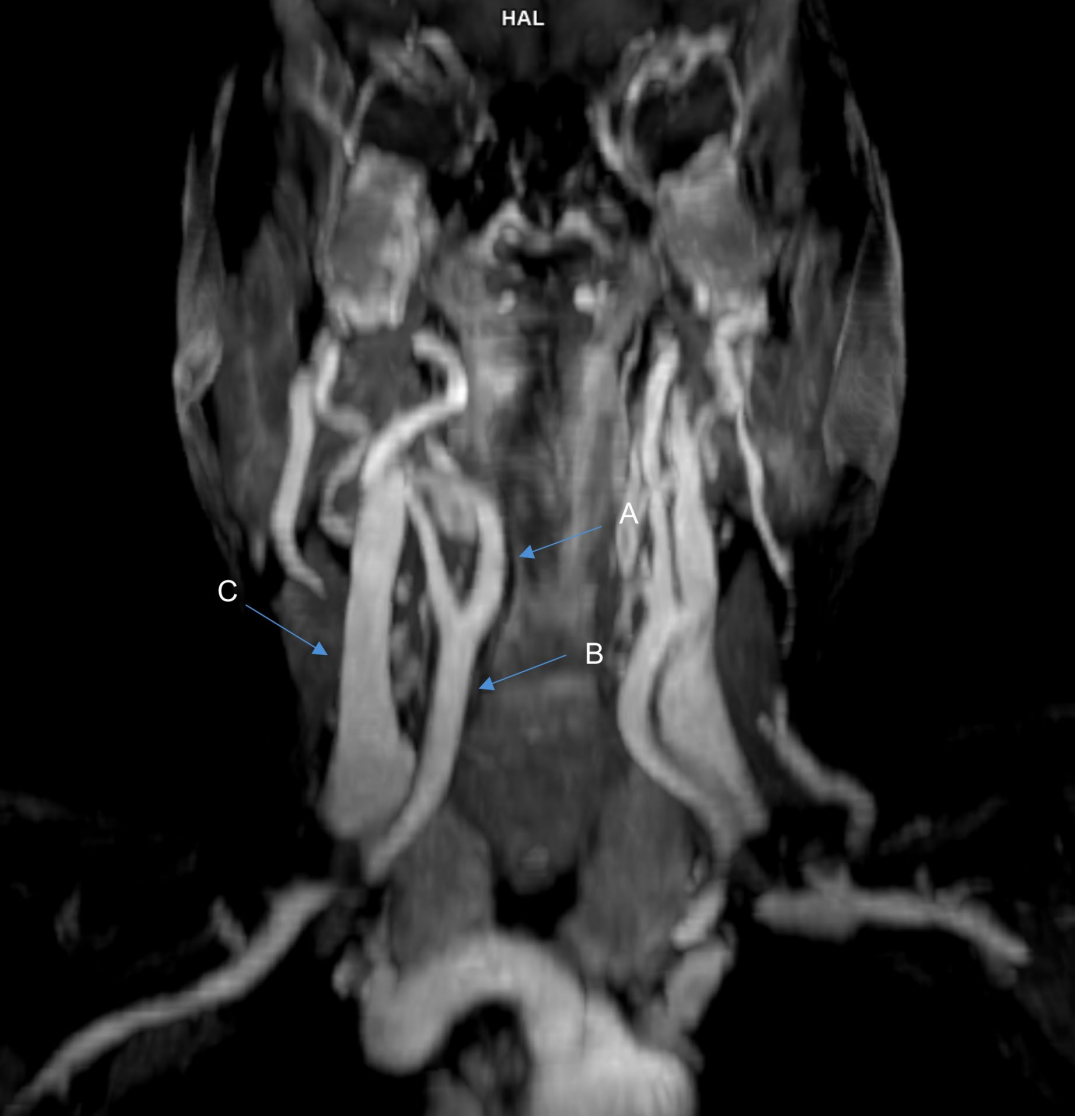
MRI, coronal view. The course of the common carotid artery and the ICA in the neck, A ICA, right-sided, B Commom carotid artery, right-sided, C Internal jugular vein, right-sided
